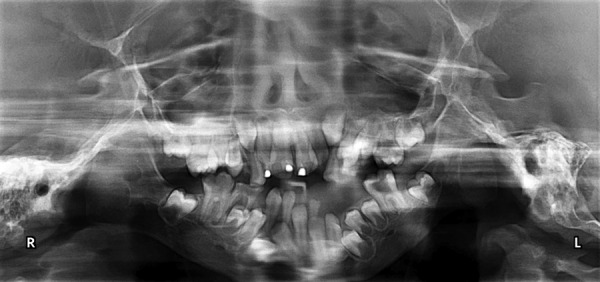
Orthopantomograph showing retained deciduous teeth, crowding, periapical lesion in relation to 46
